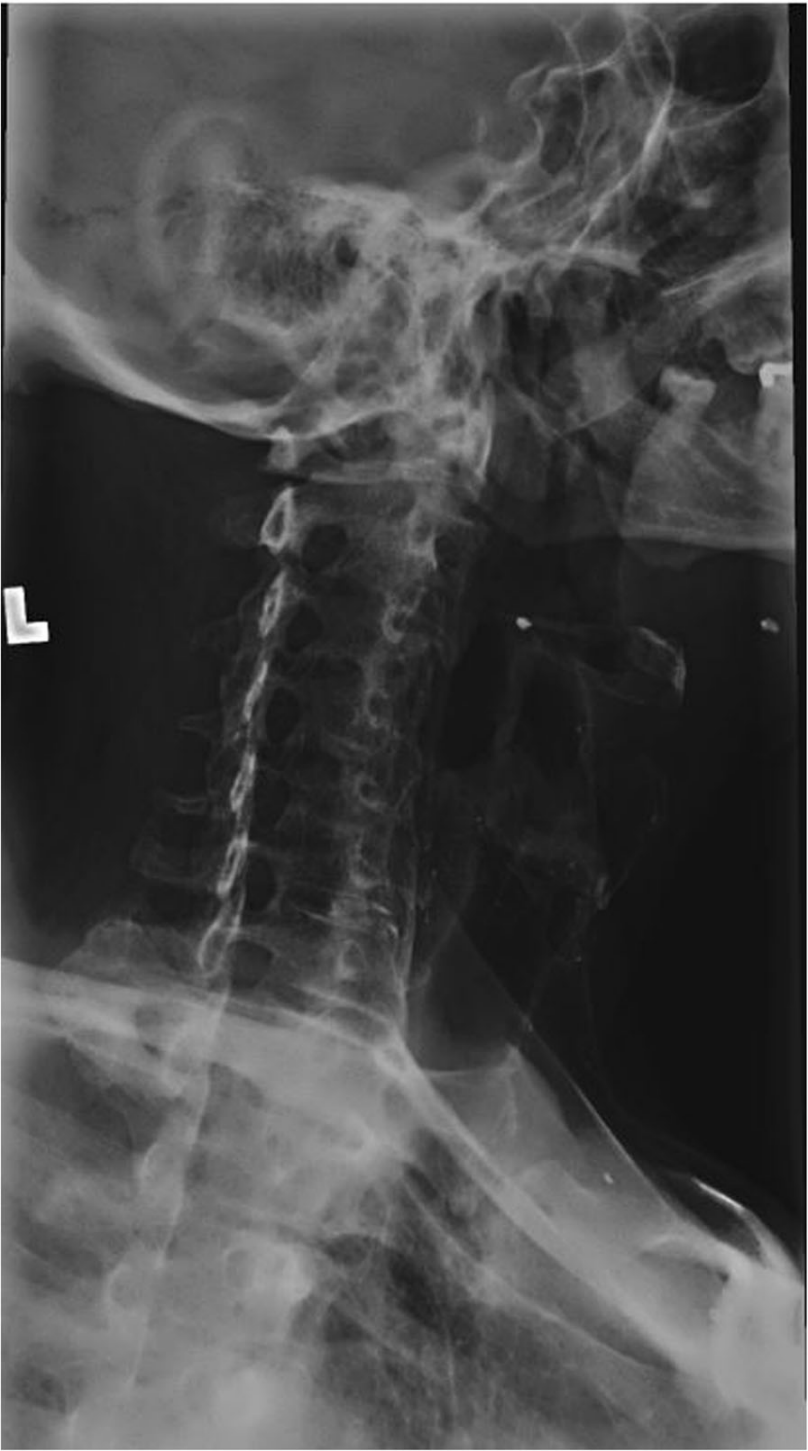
Left Oblique Plain Films, patent intervertebral foramina visualized
